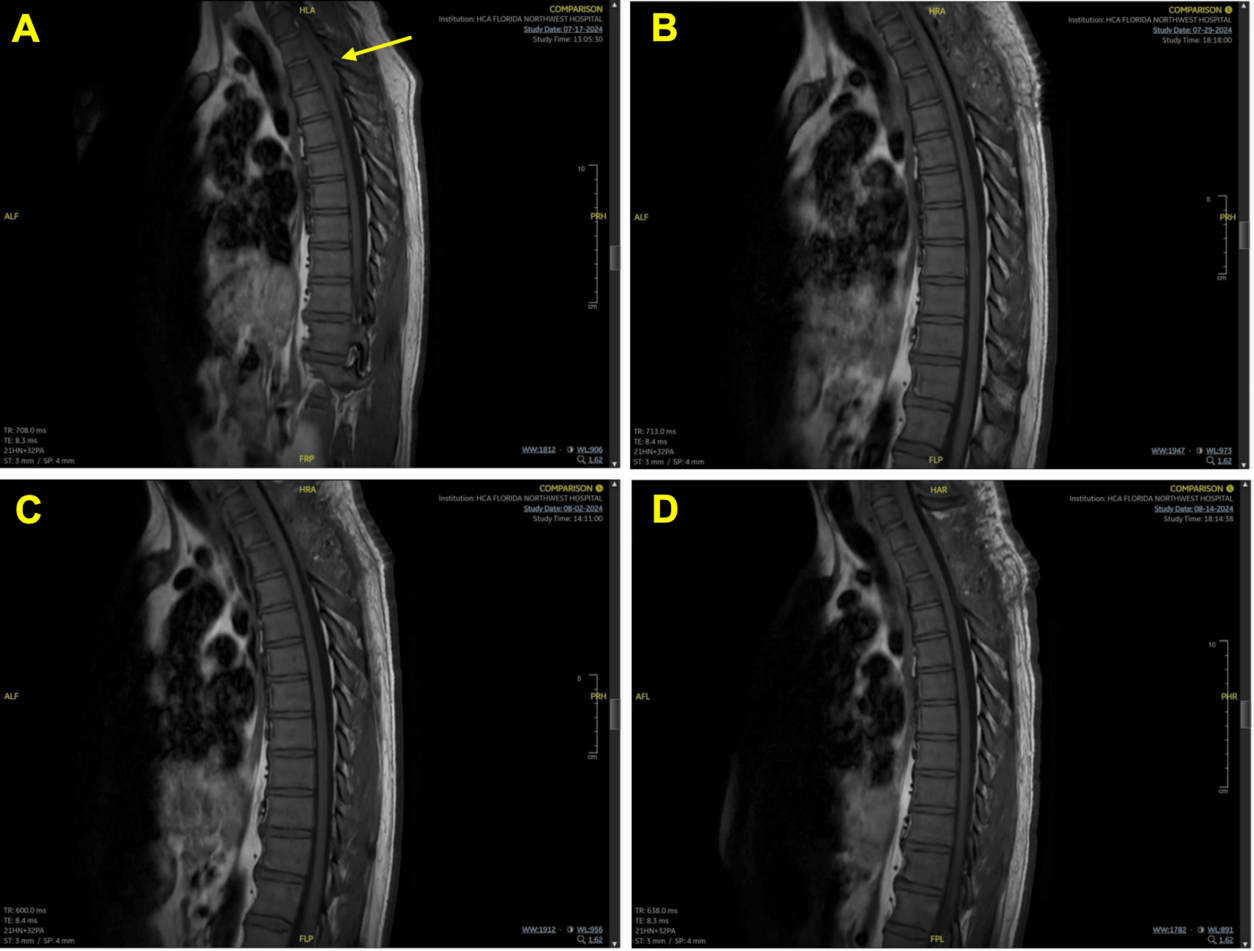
Thoracic magnetic resonance imaging (MRI) (A) Initial thoracic MRI diagnosis revealing dorsal T1-T4 spinal epidural abscess. (B-D) Post T1-T3 laminectomy MRI of the thoracic spine and interim period MRI demonstrating resolved dorsal spinal epidural abscess
